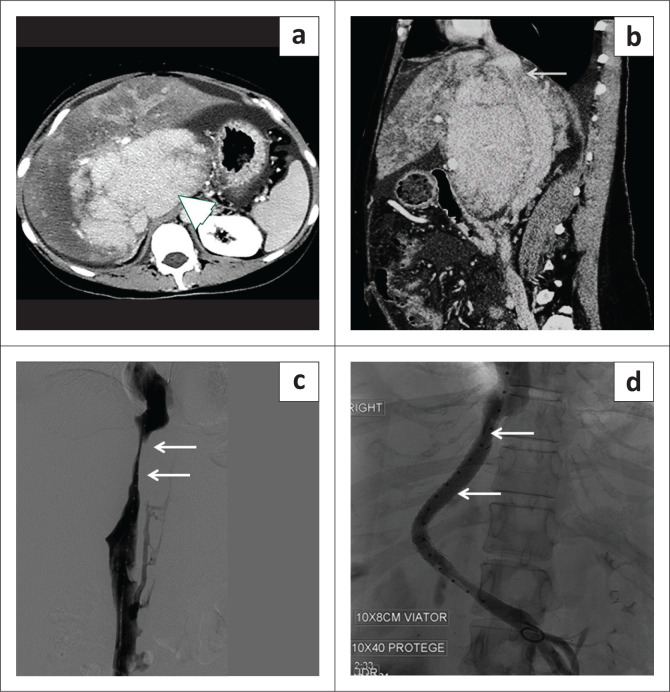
(a) Contrast-enhanced CT demonstrates preferential enhancement of the central liver of liver (white arrowhead) in a patient with Budd-Chiari syndrome. (b) Sagittal CT abdomen showing narrowing of the infrahepatic inferior vena cava (white arrowhead) and an enlarged caudate. (c) Venogram confirms the diagnosis. (d) Venogram placement of porto-systemic venous shunt.
